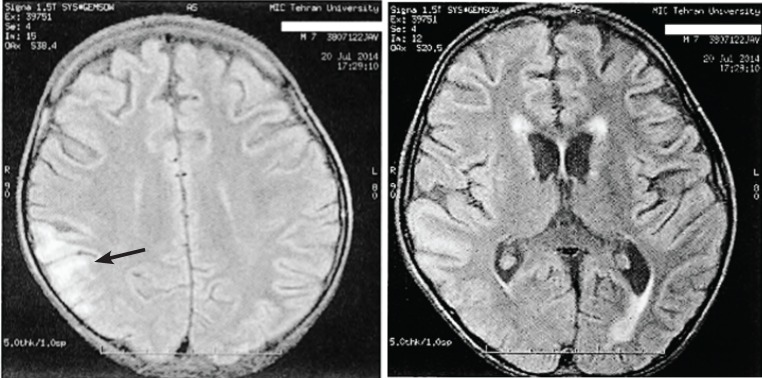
Magnetic resonance imaging (MRI) in FLAIR sequence showing ‘abnormal right parietal corticosubcortical high signal’
